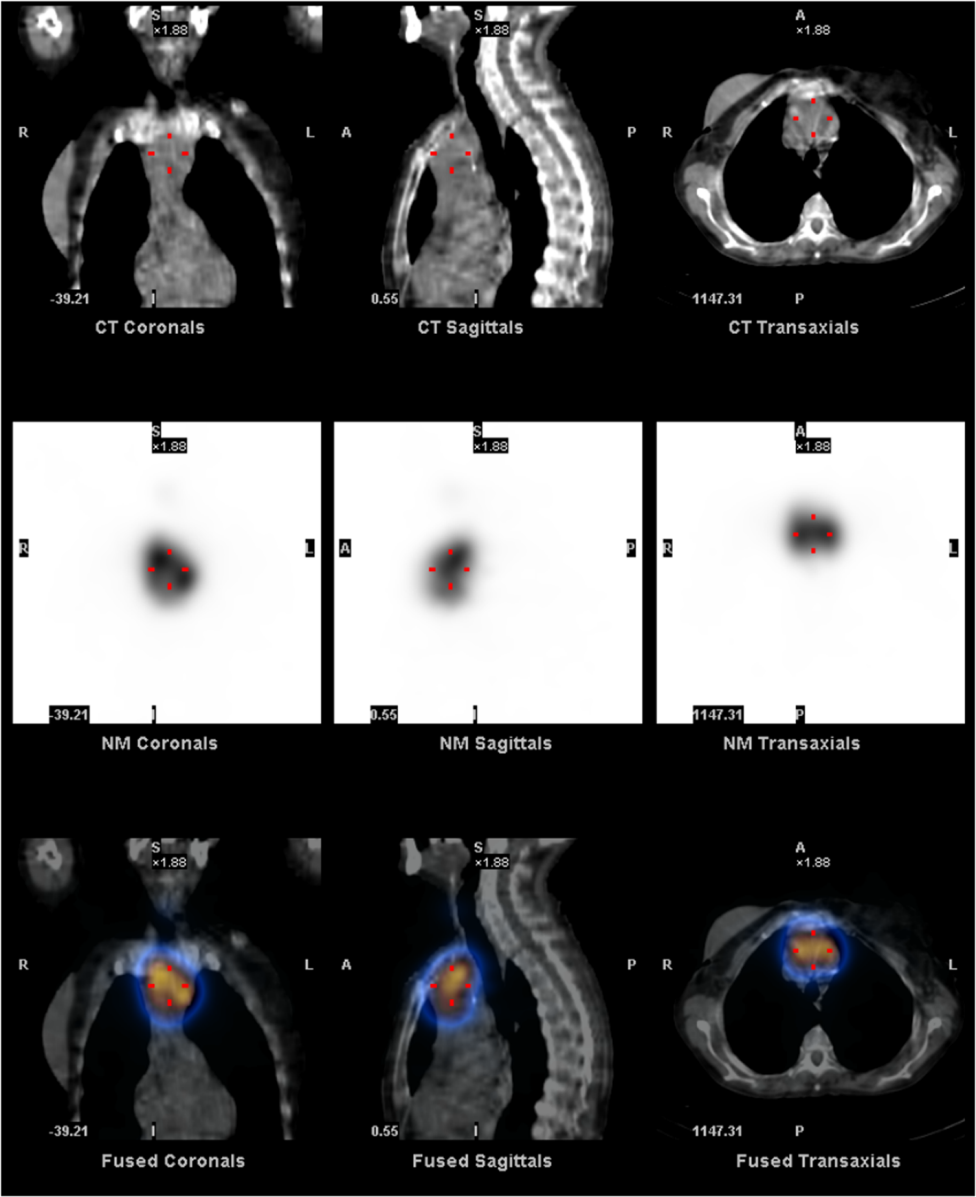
Corporal scintigraphy with I-131 with single-photon emission computed tomography showing an intrathoracic mass (60 × 40 mm) with increased radioiodine uptake
